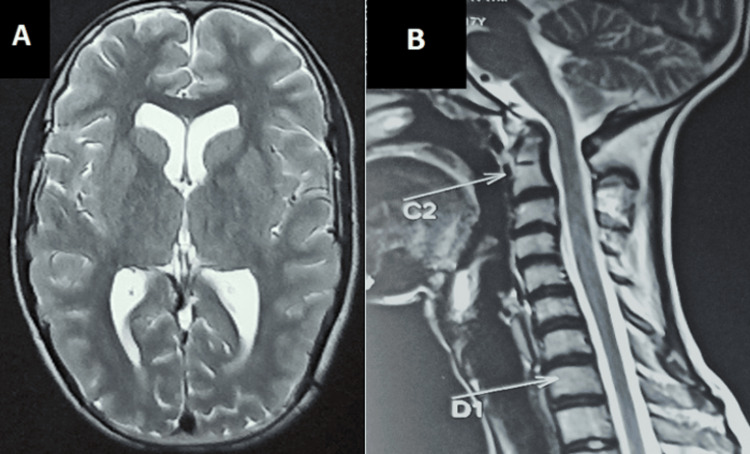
(A) MRI of the brain revealed a dolichocephalic skull with a decreased cephalic index. (B) Screening MRI of the whole spine revealed atlanto-axial subluxation with mild retroflexion of the dens, indenting the anterior subarachnoid space at the craniovertebral junction.
